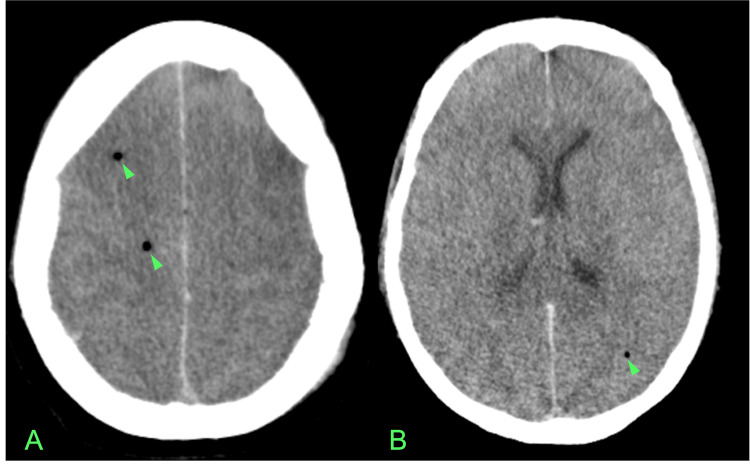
Head CT-scan after 12 hours of admission showing loss of cortico-subcortical differentiation (A), as well as effacement of the basal ganglia (B) compatible with diffuse cerebral edema. Only three small gas collections were found (arrowheads).
